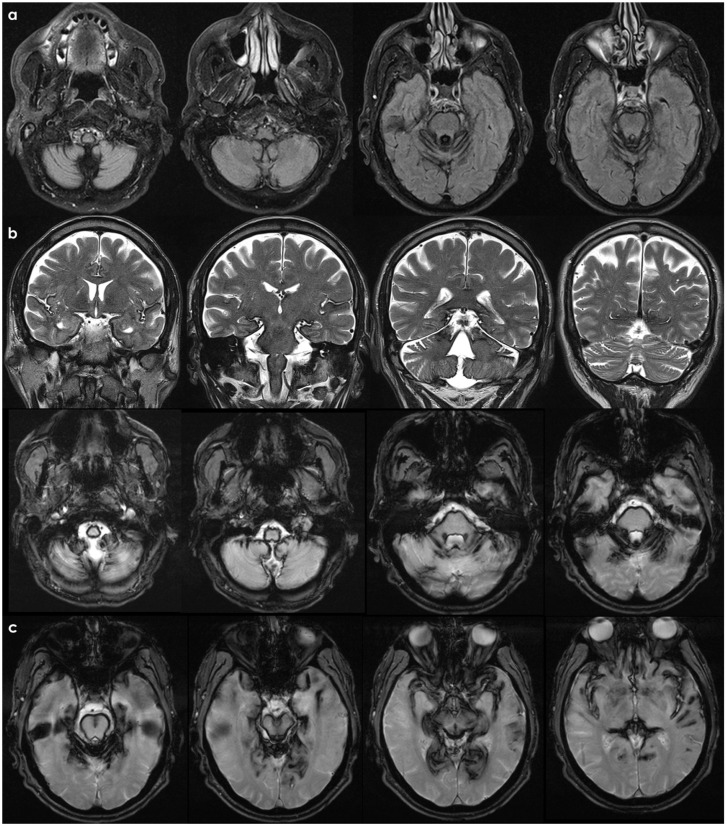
Brain MRI in the same patient of Figure 3, showing extensive, mainly infratentorial siderosis, well evident in axial FLAIR (a), coronal T2W (b) and axial T2* (c, two lines).
